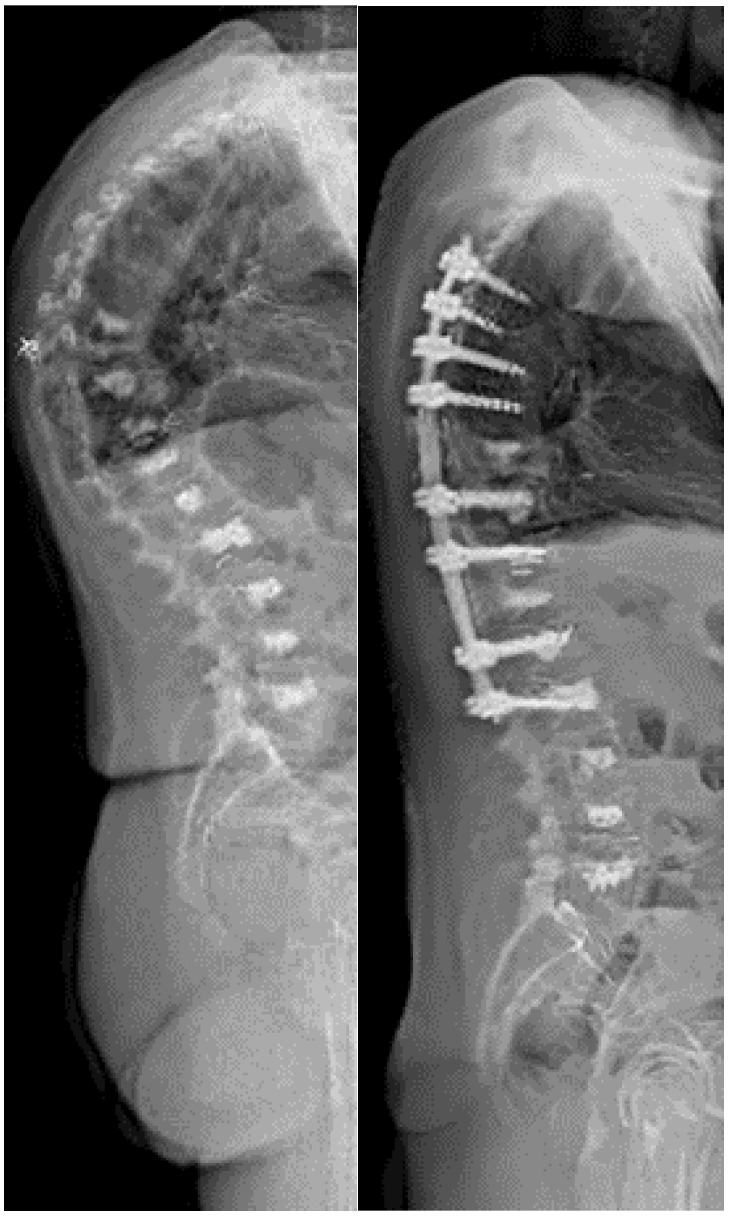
Lateral standing X-ray images before (left) and after (right) wedge osteotomy in a patient with MM with multiple vertebral lesions treated with vertebroplasty. Note the correction of the sagittal profile.
